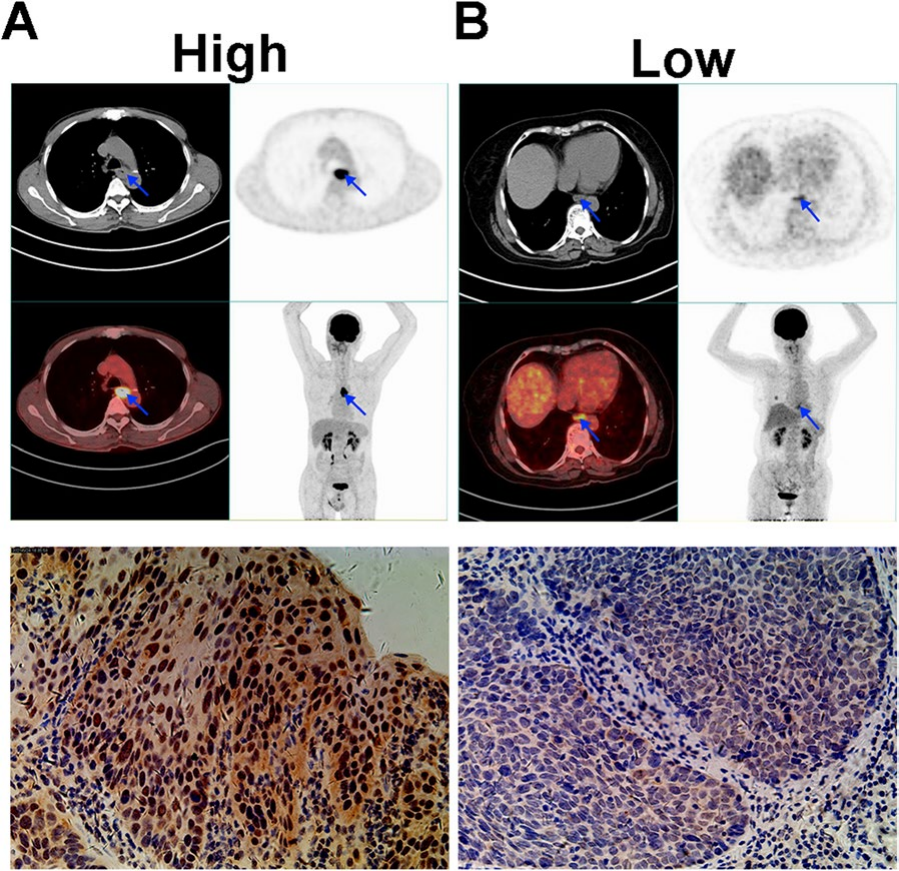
PET/CT imaging for ESCA patient with different SUV_max_, SUV_mean_, TLG and MTV. A SUV_max_ = 17.67, SUV_mean_ = 10.82, TLG = 72.29 and MTV = 7.15. B SUV_max_ = 5.64, SUV_mean_ = 3.09, TLG = 8.42, and MTV = 2.76
